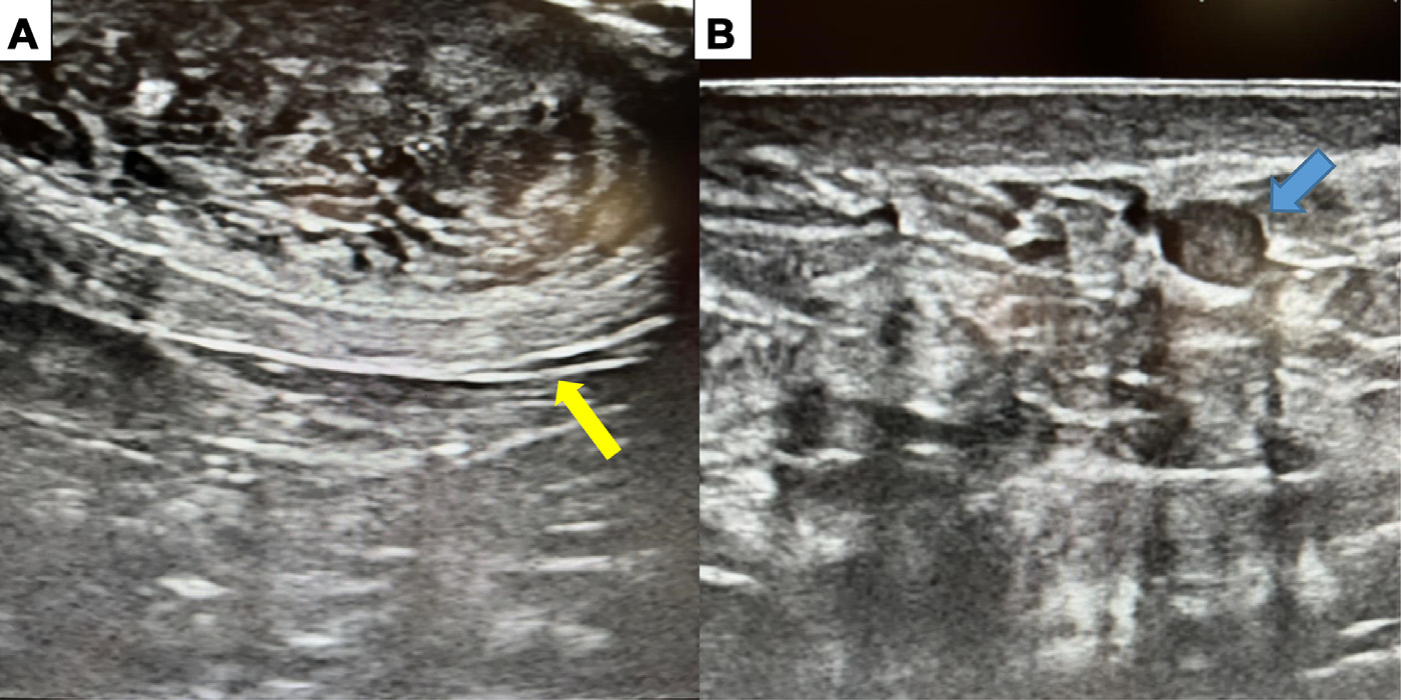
Images of a breast ultrasound of the left breast; heterogeneous and swollen appearance of the nipple (A) (yellow arrow), focal ductal dilation in the inferolateral quadrant showing a tissue-like projection (B) (blue arrow).
